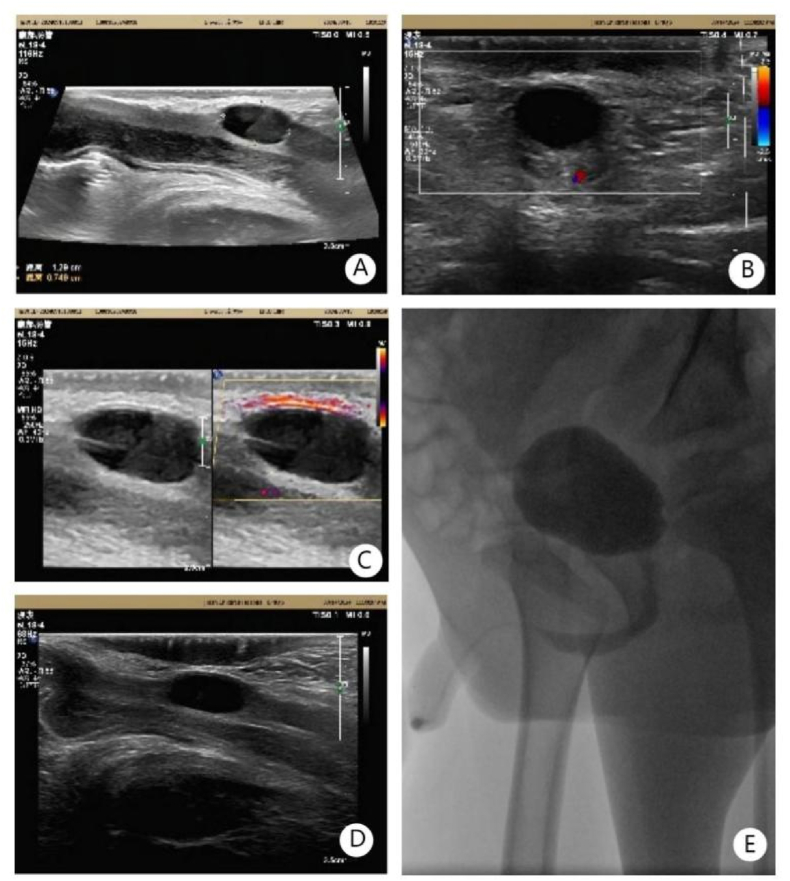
Ultrasound and retrograde cystography findings at admission. Panels A, B, C, and D show an oval-shaped cystic mass in the urethra observed on ultrasound, with clear margins, poor internal sound transmission, and visible layering; catheter echo is noted within the urethra. Panel E demonstrates retrograde cystography findings, indicating significant narrowing of the urethra anterior to the pubic bone, with slow passage of the contrast agent.
